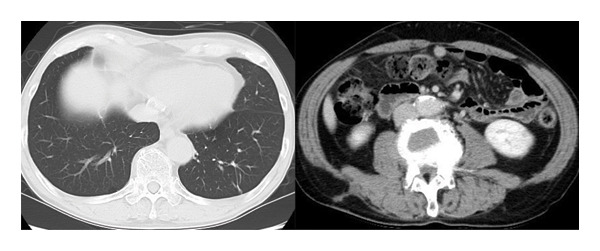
Four months after discontinuing methotrexate, CT reveals spontaneous resolution of the lung and psoas lesions.
